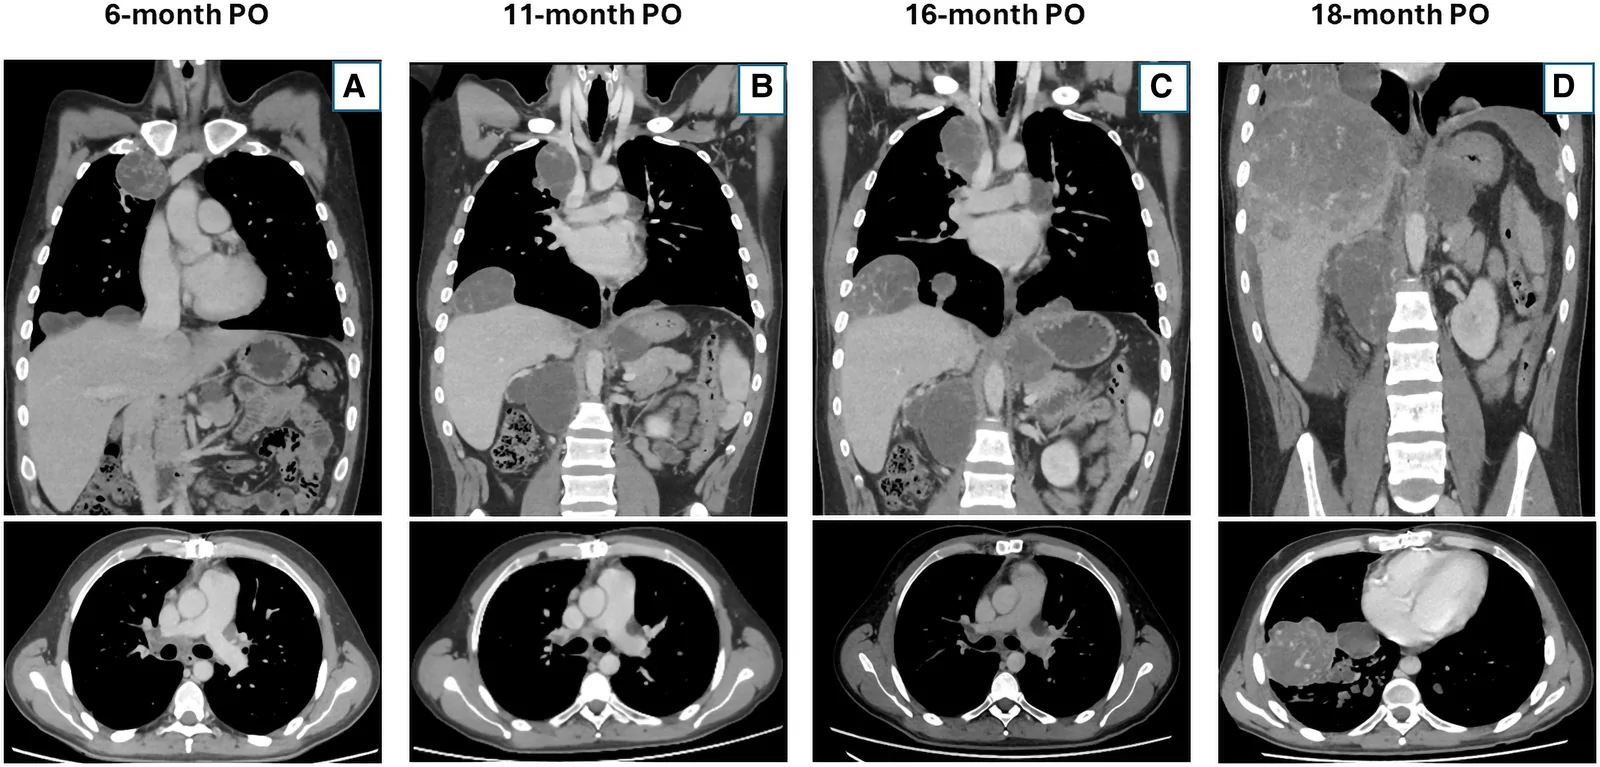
Serial post-operative chest and abdominal computed tomography scans demonstrating disease progression. (*A*) At 6 months, (*B*) 11 months, and (*C*) 16 months post-op (PO), coronal (top) and axial (bottom) views show stable post-surgical findings with no evidence of intracardiac recurrence. (*D*) At 18 months post-op, scans reveal a significant locoregional and distant disease progression, characterized by a large, heterogeneous, lobulated mass in the right lung base (arrow) accompanied by extensive hepatic and retroperitoneal metastatic lesions.
